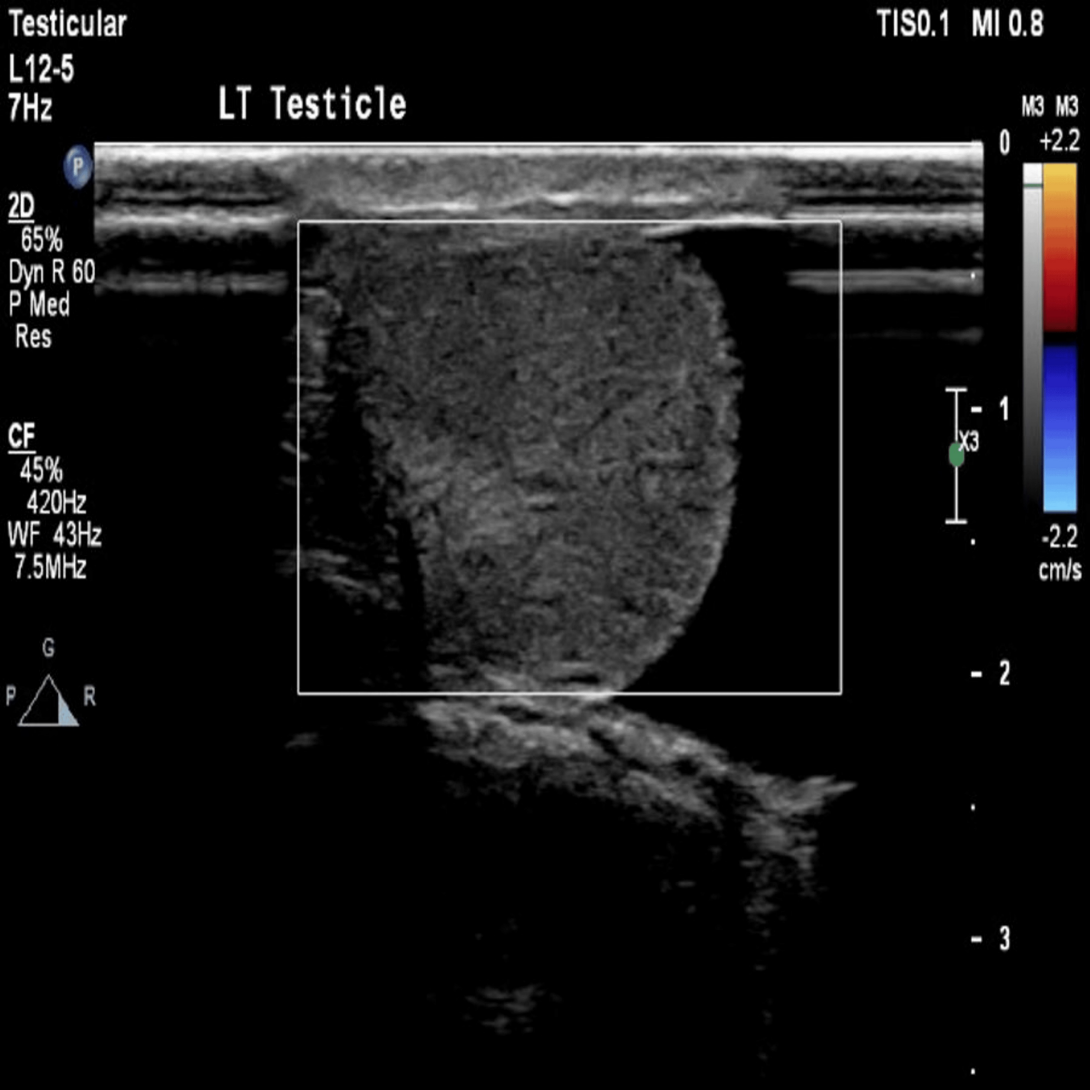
Ultrasound image of left testicle demonstrating hypoechoic relative to the right and lacks internal vascularity on color Doppler
